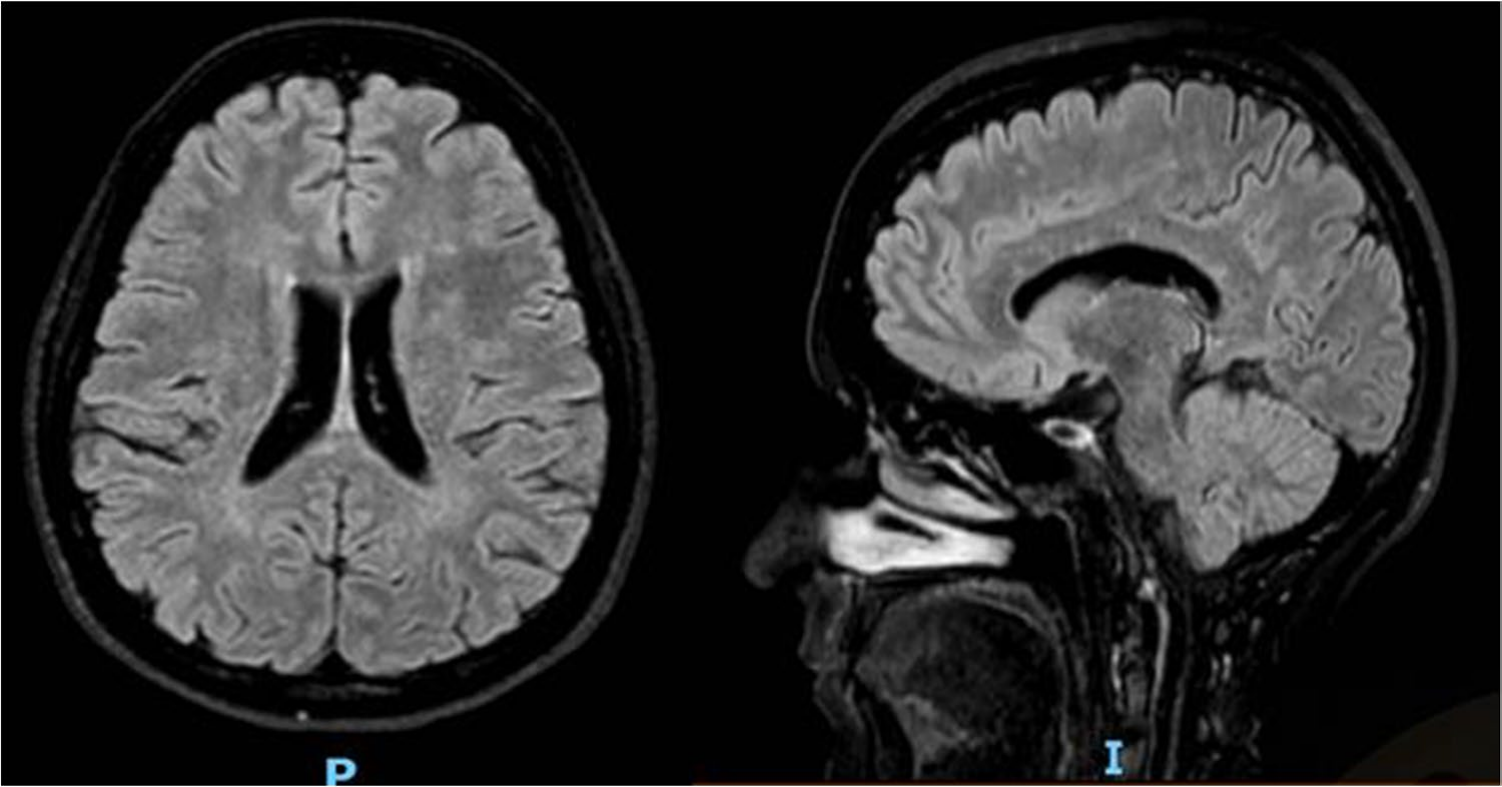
Last brain MRI, showing improvement of radiological findings
